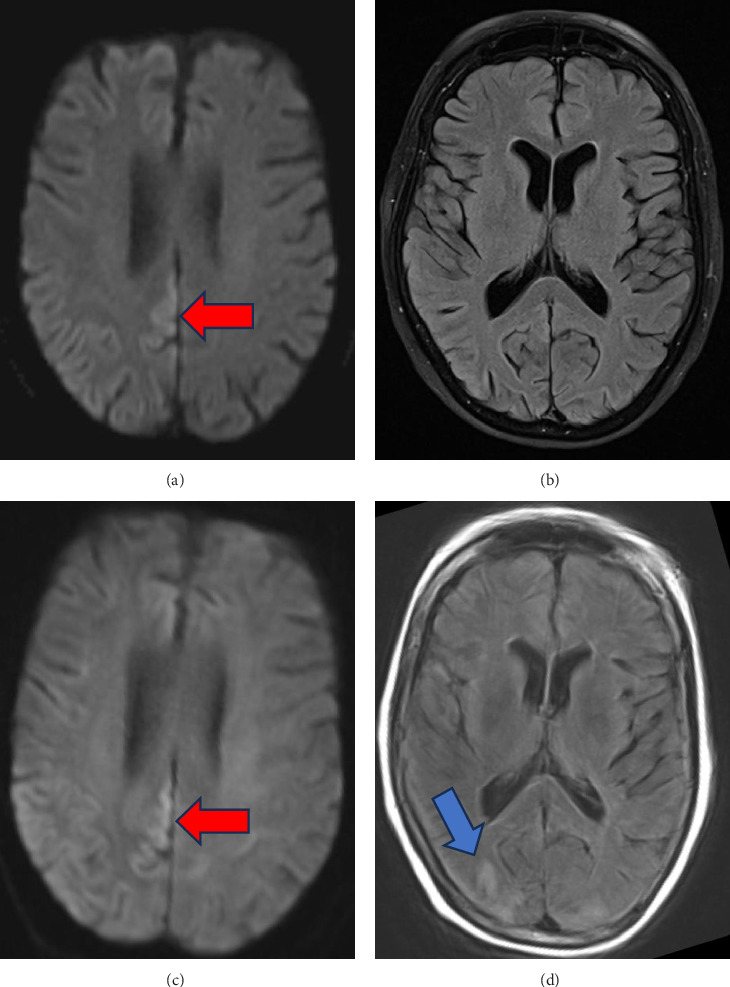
Magnetic resonance imaging of the patient's brain with and without contrast. (a) and (b) were obtained on the second week of symptoms at an outside hospital. (a) Diffusion weighted imaging (DWI) showing restricted diffusion most prominent in the right occipital lobe (red arrow). (b) T2/FLAIR sequence showing minimal FLAIR changes in that region. (c) and (d) were obtained on the third week of symptoms. (c) the DWI scan again showing diffusion restriction in the occipital lobe. In (d), progression of FLAIR signal in the right occipital lobe (blue arrow) can be observed on the T2/FLAIR sequence compared with (b).
